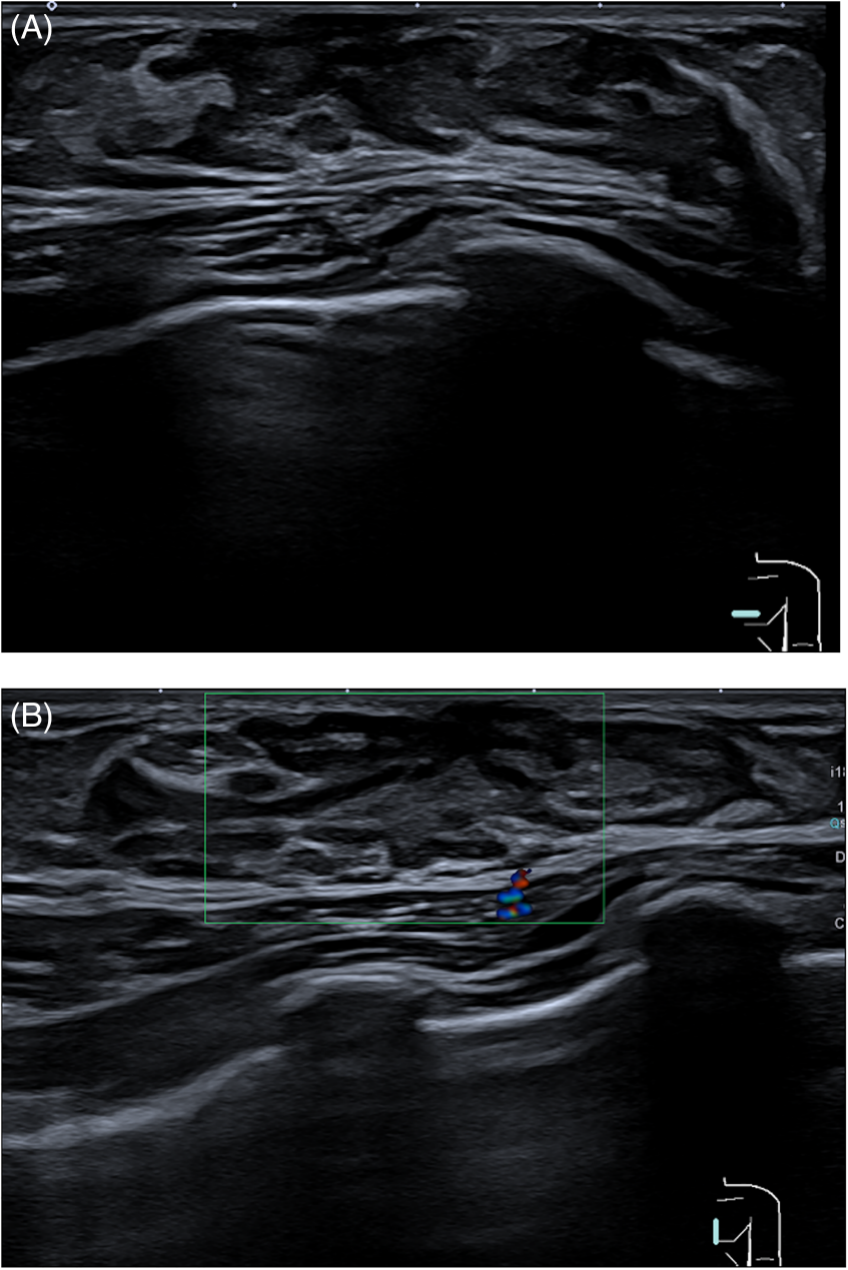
(A) B-mode and (B) color Doppler ultrasound demonstrates retroareolar hypoechoic tissue relative to fat with absence of blood flow in keeping with breast buds.
